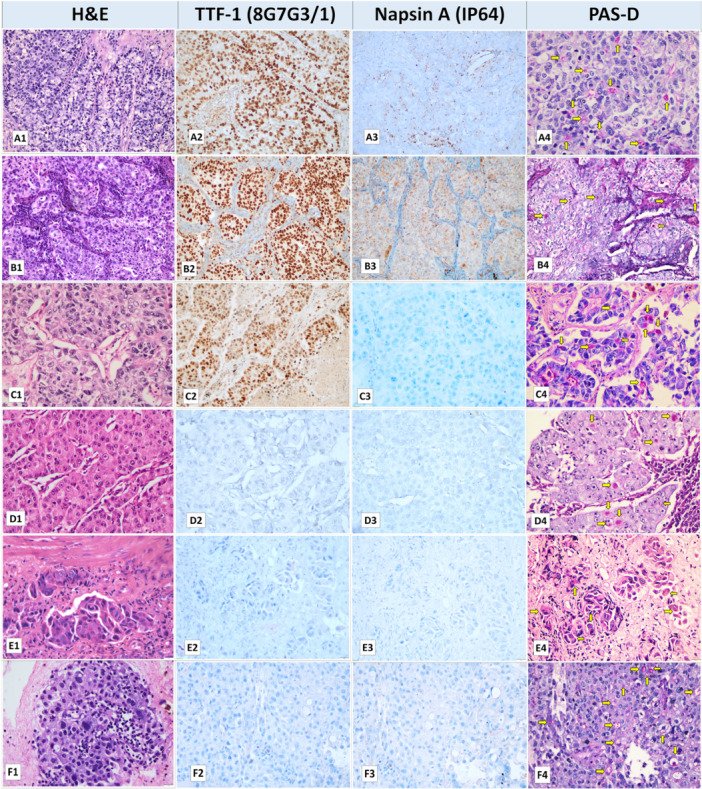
Expressions of TTF‐1, NapsinA and PAS‐D in clinically confirmed primary lung adenocarcinomas. A1‐4 and B1‐4 (Resection, x400): TTF‐1_pos_/NapsinA_pos_/PAS‐D_pos_ adenocarcinoma. C1‐4 (Resection, x400): TTF‐1_pos_/NapsinA_neg_/PAS‐D_pos_ adenocarcinoma. D1‐4 (Resection, x400), E1‐4 (Bronchoscopic biopsy, x400) and F1‐4 (Cell block of transthoracic fine needle aspiration, x400): TTF‐1_neg_/NapsinA_neg_/PAS‐D_pos_ adenocarcinoma. A4‐F4: Arrows highlighting PAS‐D_pos_ intracytoplasmic mucin droplets (x400).
